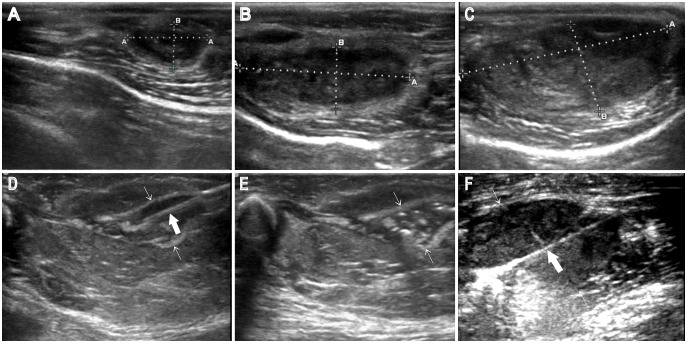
The ultrasound images of VX_2_ tumor and puncturing guidance. A, B, C: a VX_2_ tumor on ultrasound (US) at 0, 6th and 12th day after treatment, respectively; D: ^131^I-chTNT intratumoral injection; E: after ^131^I-chTNT intratumoral injection; F: radiofrequency ablation (RFA). Thin arrow: the VX_2_ tumor; thick arrow: PTC needle (D) or RFA electrode (F).
